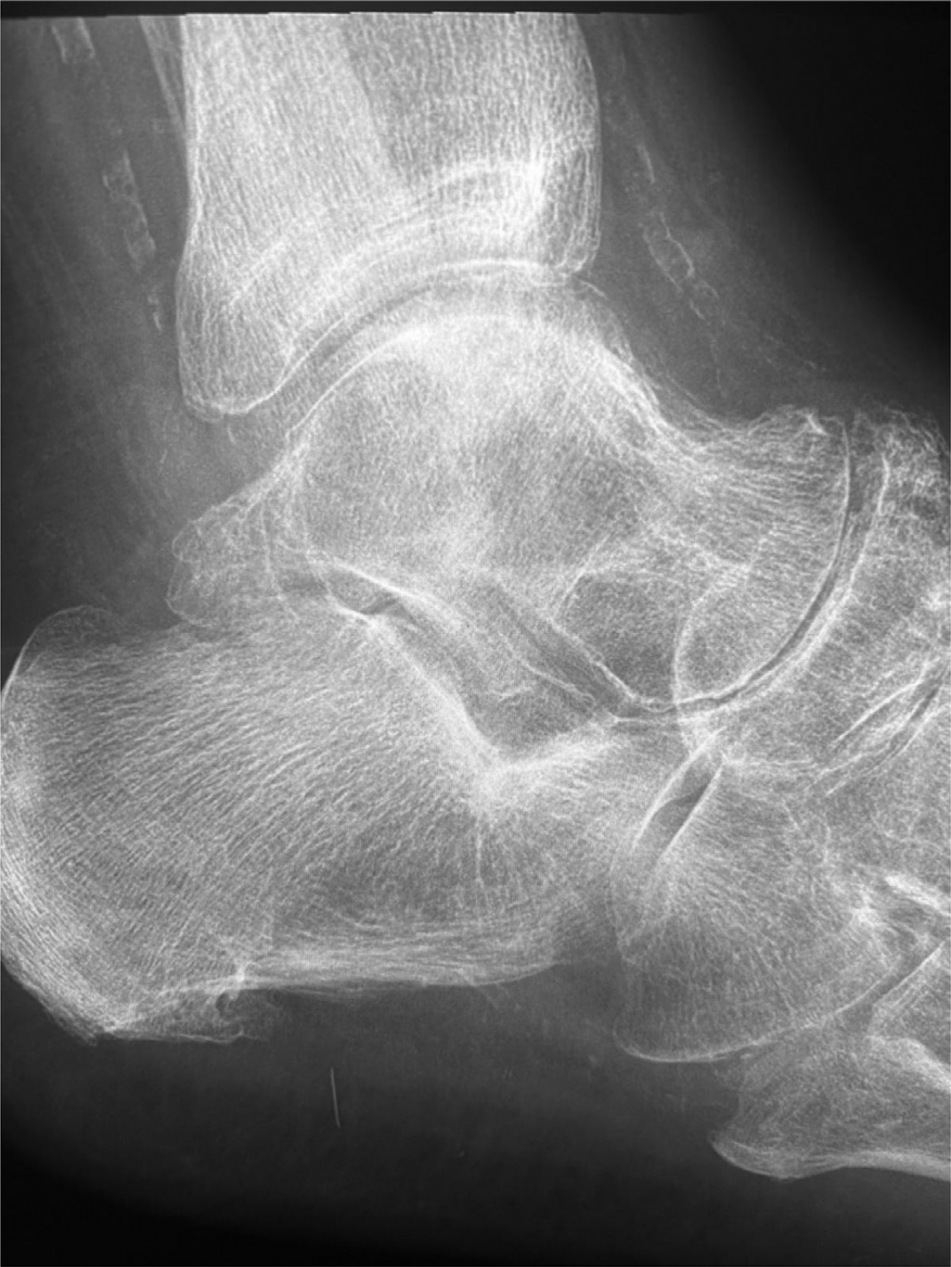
X-ray of the ankle of a patient with long-standing type 1 diabetes and peripheral neuropathy, showing bone resorption and fragmentation especially in the dome of the talus (osteonecrosis). Note the calcification of the tibialis posterior and the dorsalis pedis arteries.
